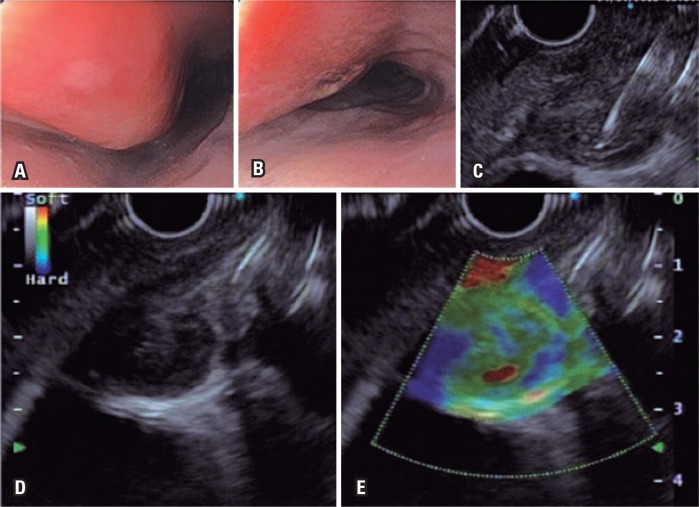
High digestive endoscopy and echoendoscopy. (A and B) Lateral wall bulging of esophagus wall, with elevated fusiform injury and erosion; (C) Echoguided puncture of mediastinal lymph node; (D) Hypoechogenic lymph node, heterogenous, with hyperechogenic center, and hypervascularization showed in Doppler ultrasound (high flow) measuring around 15mm; (E) Qualitative elastography with soft characteristics (green-like)
